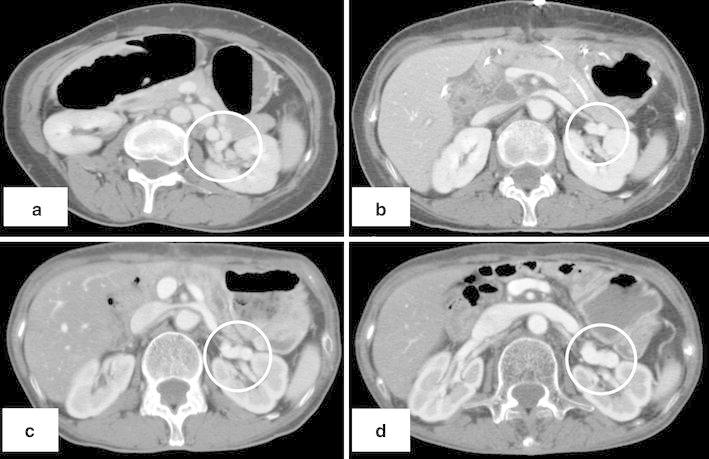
Review of abdominal computed tomography scans. a Preoperatively, b 6 months postoperatively, c 1 year postoperatively, d 2 years and 2 months postoperatively. The shunt vessel gradually enlarged after pancreaticoduodenectomy (*circle*)
